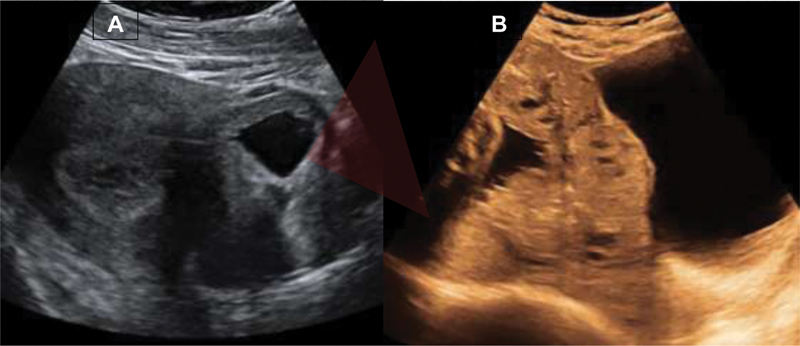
A. Sagittal first trimester transabdominal ultrasound image of a Cesarean scar pregnancy highlighting the “high-risk-for-placenta accreta spectrum triangle” (implantation on the lower anterior quarter of the uterus, and into the Cesarean scar niche); B. Sagittal third trimester transabdominal ultrasound image of a placenta accreta spectrum disorder on a placenta previa completely covering the cervical internal os (arrow).
